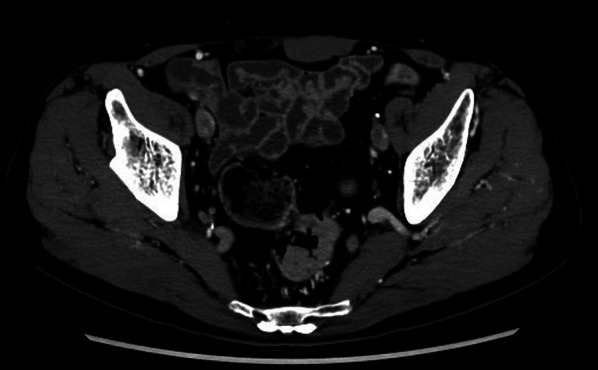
Abdominal contrast-enhanced computed tomography (CT) revealed a thickened rectal wall, but no obvious distant metastasis (white arrow)
